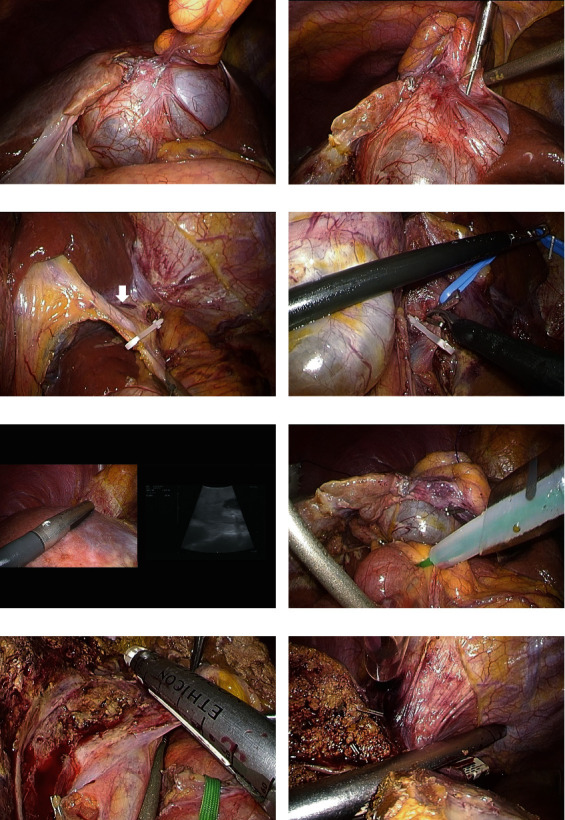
Surgical method is shown. A regrowth tumor was observed in the medial segment of the liver (a). Aspiration of cystic fluid was performed to secure a field of view (b). The left hepatic artery (white arrow) was diverged from the left gastric artery and clipped (c). The left portal vein was identified and divided after clipping (d). The transection line was set using intraoperative ultrasonography (e). Pringle's maneuver was performed prior to hepatic parenchyma transection (f). The (g) left hepatic duct and the (h) left hepatic vein were divided by a linear stapler.
